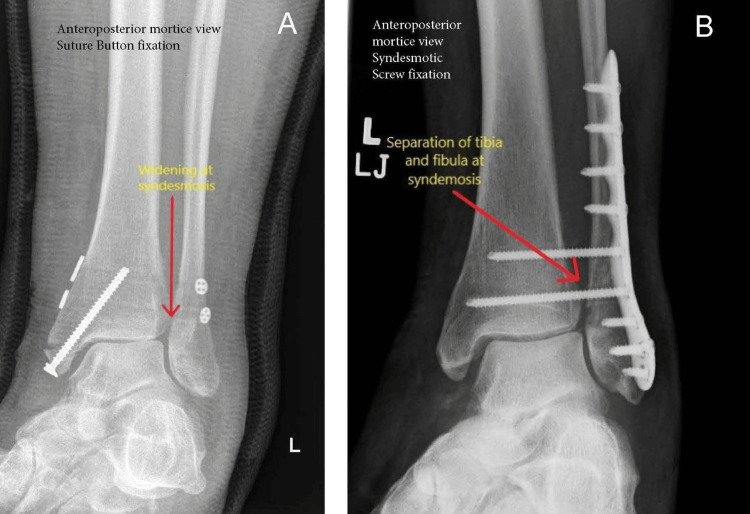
Plain radiograph showing widening of the increased separation of the tibia and fibula following fixation with the suture button device (A) & syndesmotic screws (B), indicating failure of fixation
